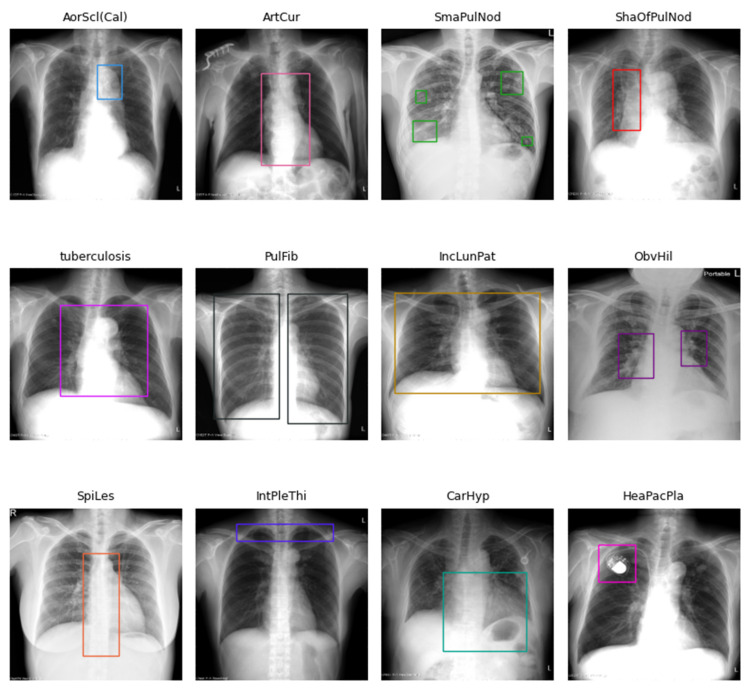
Examples of X-ray images and their corresponding bounding boxes across the 12 disease categories.
